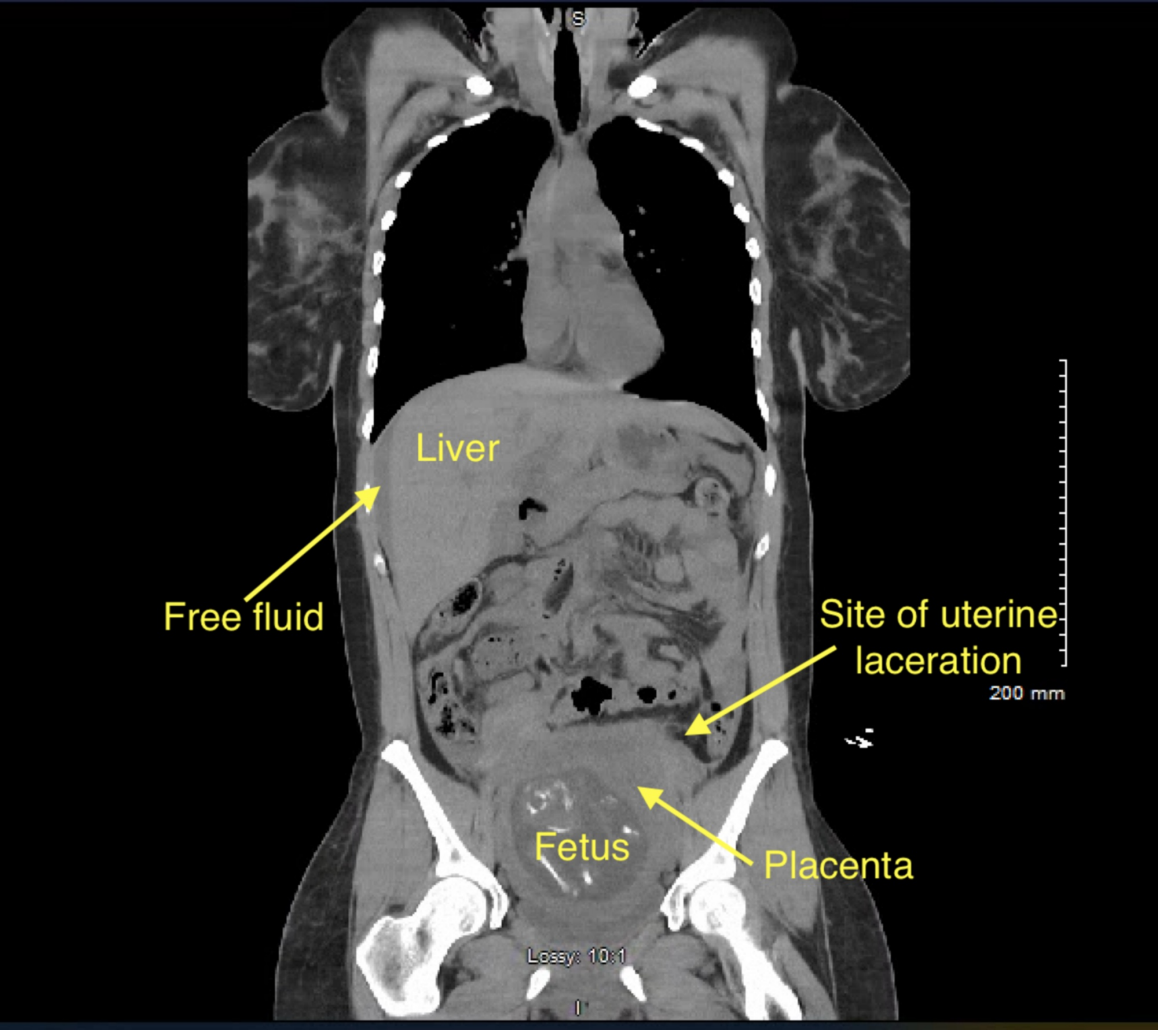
Coronal reconstruction of CT from Figure 1, also with free intra-peritoneal free fluid.
